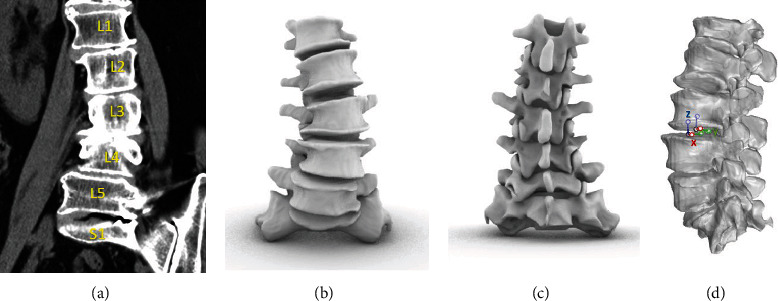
(a) Digitized contours of lumbar vertebrae in coronal plane. (b, c) Three-dimensional anatomic vertebral model constructed from the computed tomography. (d) Anatomic coordinate system to measure kinematics of the endplates.
